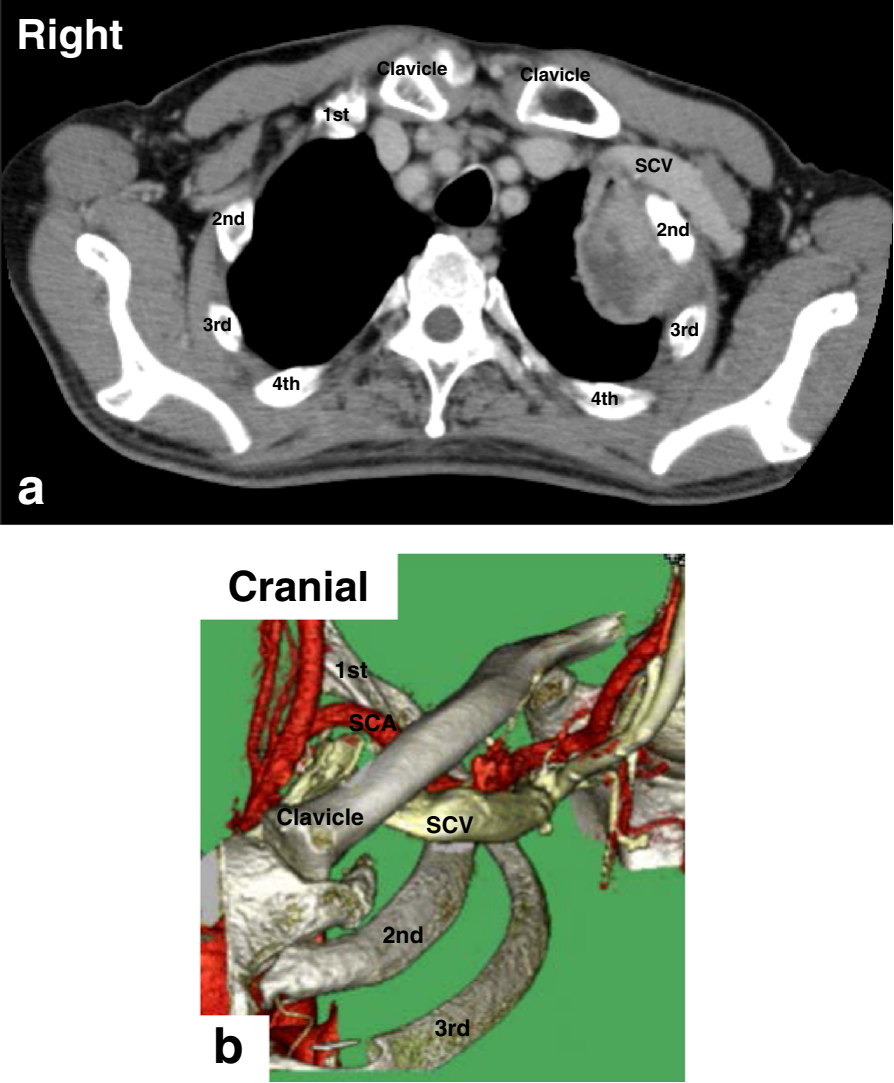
a Computed tomography (CT) showed the tumor infiltrating the chest wall mainly surrounding the second rib. The subclavian vein (SCV) ran between the clavicle and the second rib. b Three-dimensional (3D) CT clearly demonstrated the structure of thoracic apex consisted with the shorter first rib, SCV and the clavicle. The first rib did not reach the manubrium of the sternum
